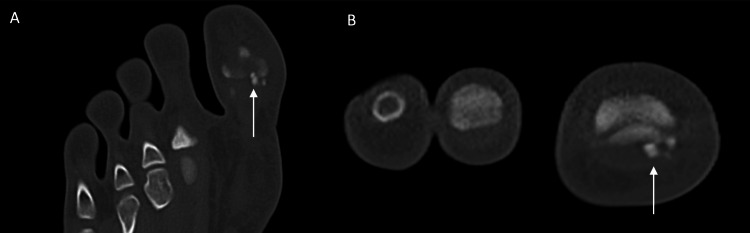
CT coronal (A) and axial (B) images showing sclerosis of subhallucal sesamoid bone (white arrows)
